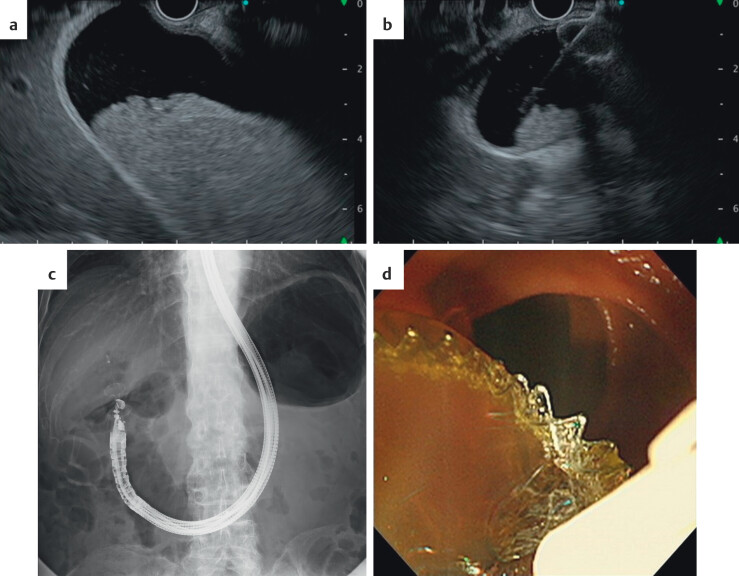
Endoscopic ultrasound-guided gallbladder drainage with one-step
